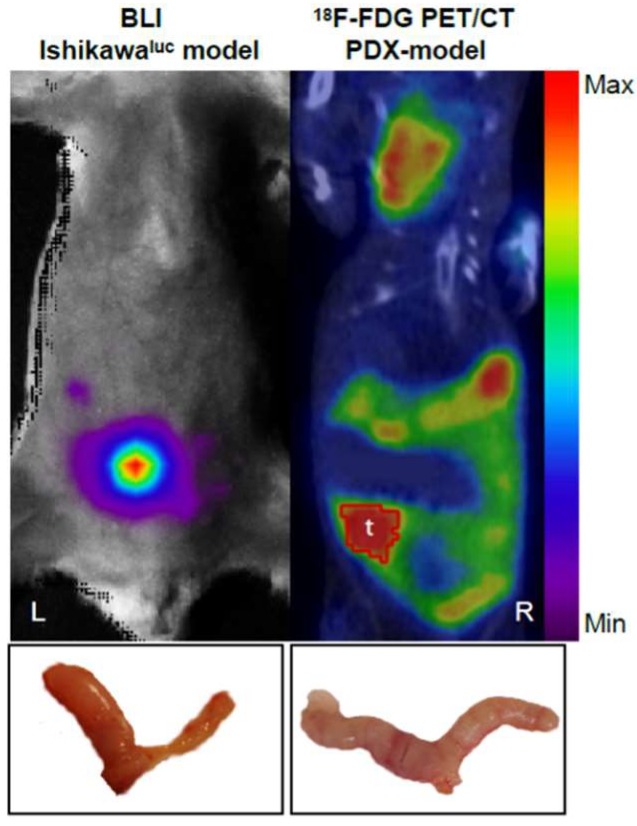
Comparison of imaging techniques for tumour evaluation. Bioluminescence imaging (BLI) enables the monitoring of cell-line-based orthotopic endometrial carcinoma, here demonstrated in a xenograft model generated from luciferase expressing Ishikawa cells (L). 18F-FDG PET/CT imaging is well suited for detection of endometrial carcinoma in PDX-models (R). Uterine tumours were confirmed by necropsy for each respective model (bottom).
